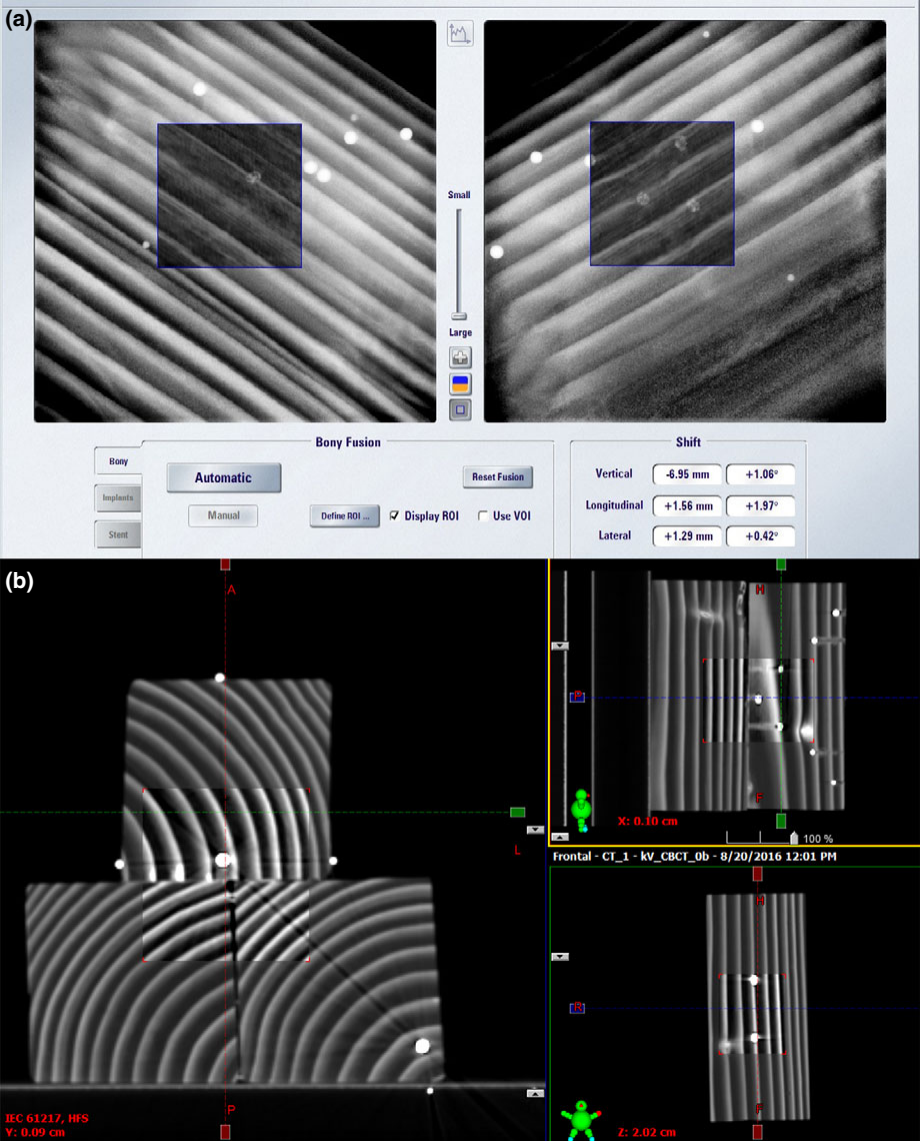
Example alignment image on the ExacTrac system (a) and on the CBCT system (b). The planned image is in the spyglass window in each.
